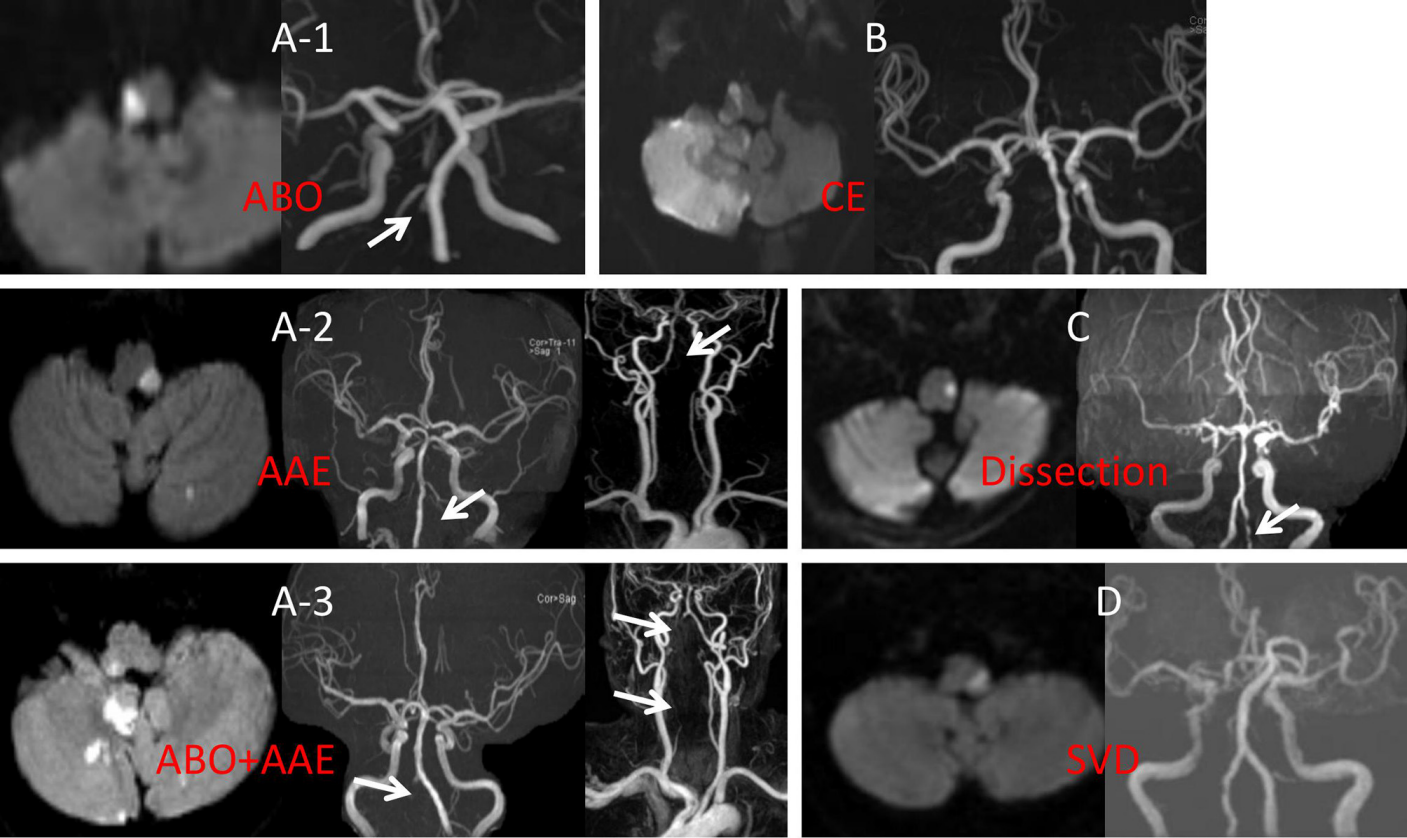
(A-1) DWI showed right caudal medullary infarction, MRA showed right intracranial VA occlusion. (A-2) DWI indicated left middle medullary and cerebellar infarction, MRA showed occlusion in the proximal left VA with no distal VA disease. (A-3) DWI showed right rostral medullary and cerebellar multiple infarction, MRA showed in both distal and proximal right VA. (B) DWI showed the right rostral medullary and cerebellar multiple infarction, MRA showed bilateral VA was normal. (C) DWI showed caudal medullary infarction, MRA showed the pearl, and string sign of left VA. (D) DWI showed middle medullary infarction, MRA showed the bilateral VA were normal. ABO, atheromatous branch occlusion; AAE, artery-to-artery embolism; CE, cardiogenic embolism; SVD, small vessel disease; DWI, diffusion weighted imaging; MRA, magnetic resonance angiography; VA, vertebral artery.
